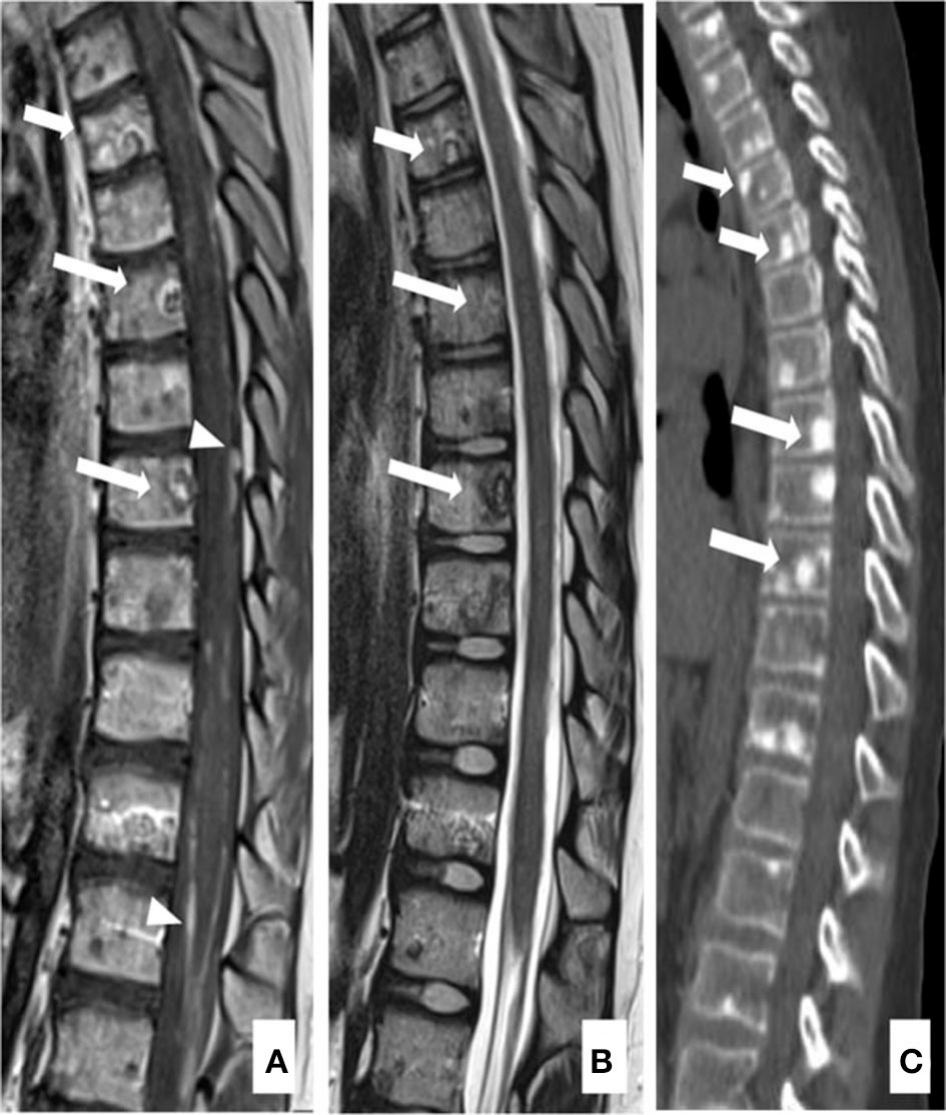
Computed tomography (CT) and magnetic resonance imaging (MRI) of bone metastases of case 1: MRI and CT exams, at 5 months after the diagnosis of DIPG. T1W after gadolinium administration (A) and the T2W (B) MRI sagittal images show diffuse leptomeningeal peri medullary contrast enhancement nodules [(A), arrowheads] and multiple and diffuse nodules of all the vertebrae with irregular and partial post-contrast enhancement on T1 images [(A, B) arrows]. The reformatted sagittal CT images confirm these hyperdense, osteoblastic, rounded lesions involving the vertebrae [(C), arrows].
